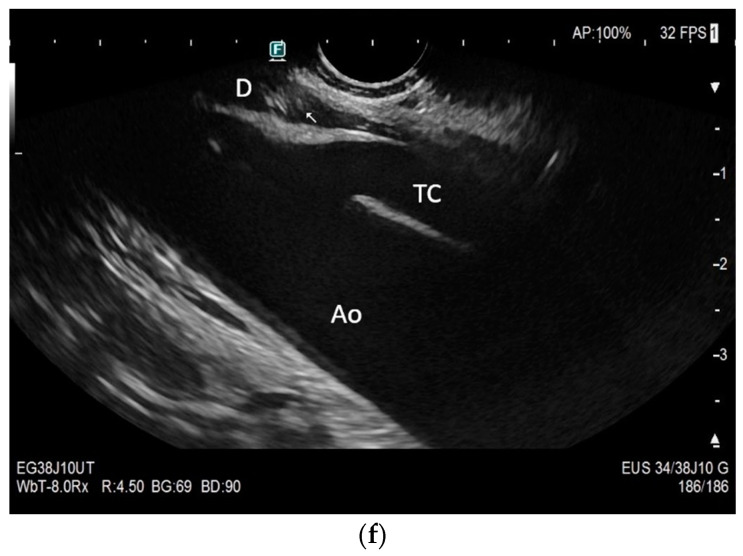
Subxiphoid transducer positions. The right diaphragmatic fat limb runs between the inferior vena cava and the aorta to the right of the spine. The left diaphragmatic limb is shown to the left of and dorsal to the aorta. Diaphragm between the markings (a). The diaphragm in the color Doppler imaging (CDI) between the vessels. The incisions should not be confused with lymphomas (b). The diaphragm on the right between the inferior vena cava and the spine (between the markings) and in front of the abdominal aorta (c). The thickened diaphragm during inspiration (between the markings) in the same position. Under optimal examination conditions, the contraction can also be visible in this position (d). The diaphragm between the aorta and liver is visible in longitudinal section (e). Endosonography shows the diaphragmatic limb ventral to the aorta (arrow) (f). Ams—superior mesenteric artery, Ao—aorta, Ard—right renal artery, D—diaphragm; IVC—vena cava inferior, PV—portal vein, TC—celiac trunk, Vl—splenic vein.
